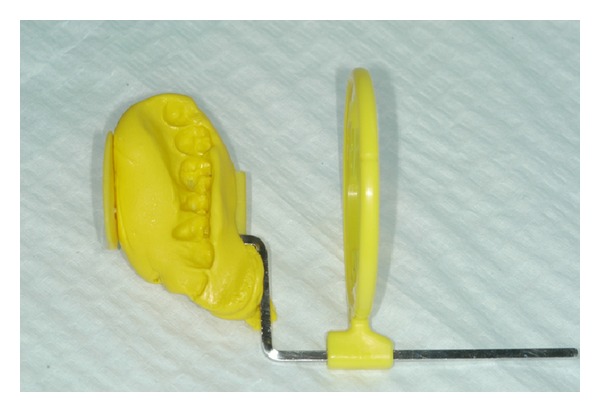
Standardized periapical radiographs were obtained using the Rinn alignment system with customized silicone bite.
